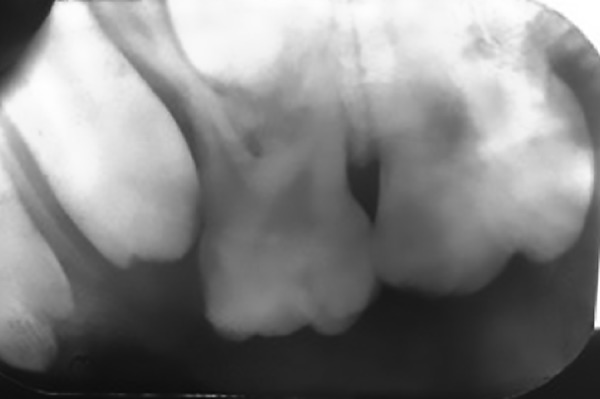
Preoperative radiograph of lesion
